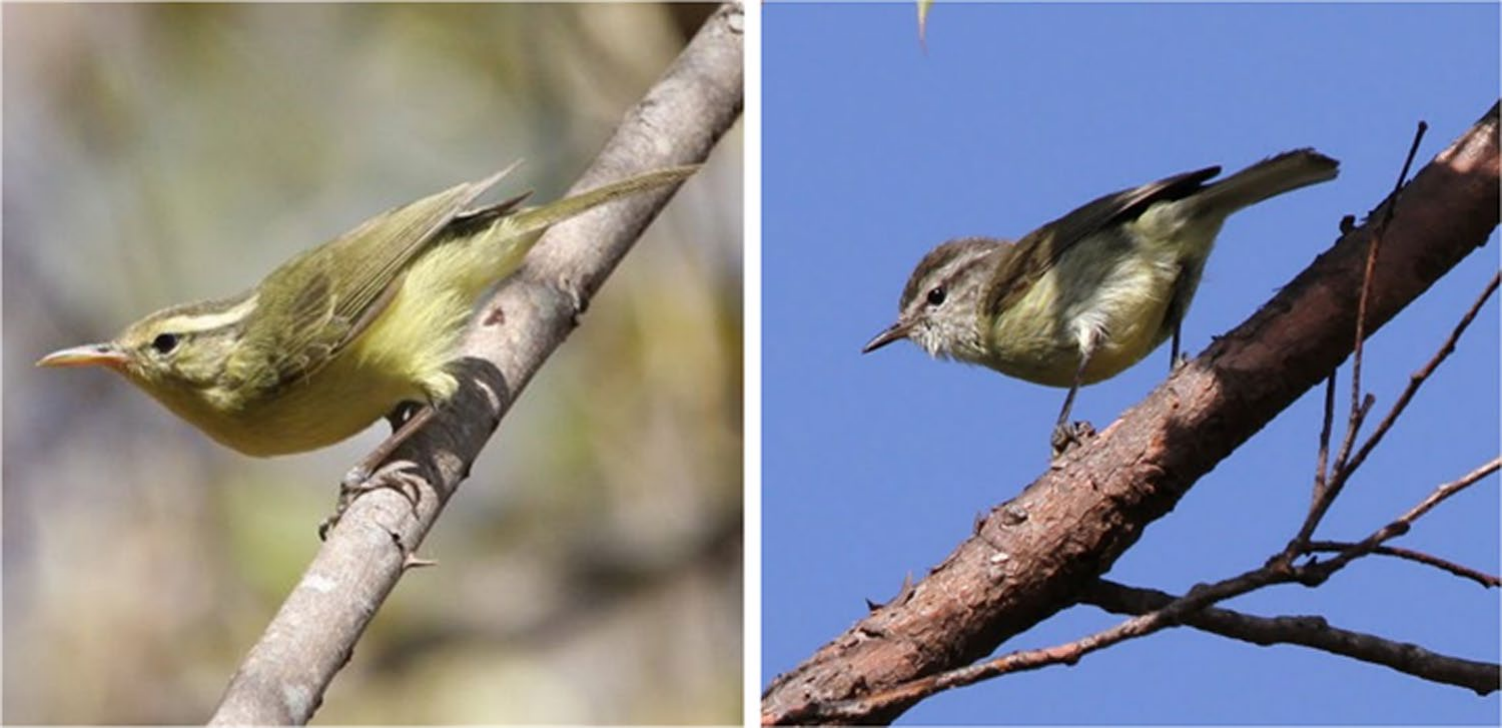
Pictures from the field of the newly described Rote Leaf Warbler (on the left; PV) and the Timor Leaf Warbler *P*. *presbytes* from Mount Ramelau in Timor Leste (on the right; CT). The unusually long, colourful bill and overall more saturated yellowish plumage of the Rote Leaf Warbler are apparent.
